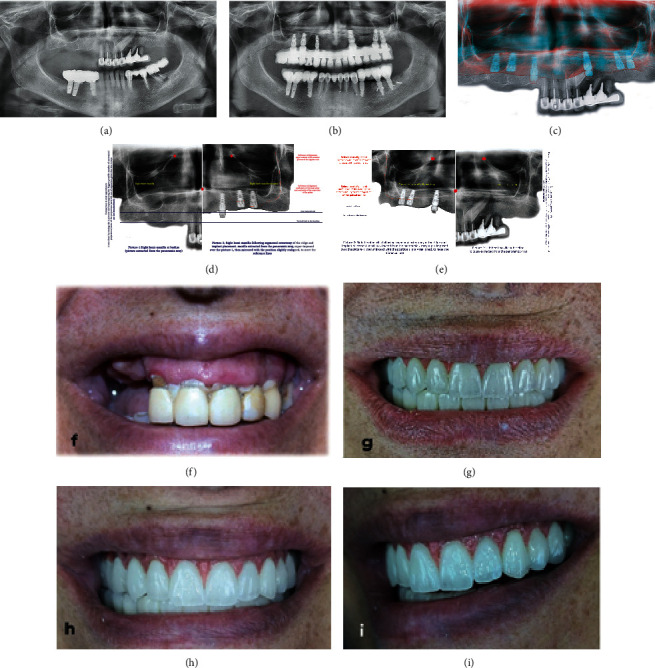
Comparisons. (a, b) Panoramic x-rays at the baseline and after case completion, respectively. (c) 2 overlayed maxillary x-rays, extracted from their respective panoramic (before and after segmental osteotomy) and superimposed to show the approximate difference between the baseline and the actual vertical level of the maxillary process. (d, e) Comparison between the baseline and after osteotomy showing the vertical translation of the maxilla in an upward direction. (f, g) Pictures revealing maximum smile at the baseline and after case completion, respectively. (h, i) Additional pictures showing the final outcome.
